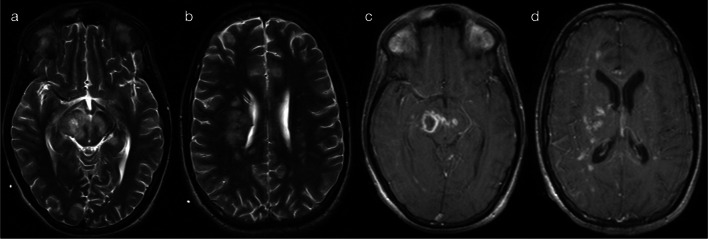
T2 hyperintensity in posterior fossa—diffuse brainstem with longitudinal tract involvement (Pattern 6B). Follow-up MRI after 2 weeks (same patient as Fig. 17 with biopsy-proven neuromelioidosis) showed T2 hyperintensities (a, b) following longitudinal white matter tracts into the supratentorial brain. Post-contrast T1-weighted imaging (c, d) demonstrated a rim-enhancing abscess in the right cerebral peduncle and multiple enhancing foci in the supratentorial brain (representing micro-abscesses)
